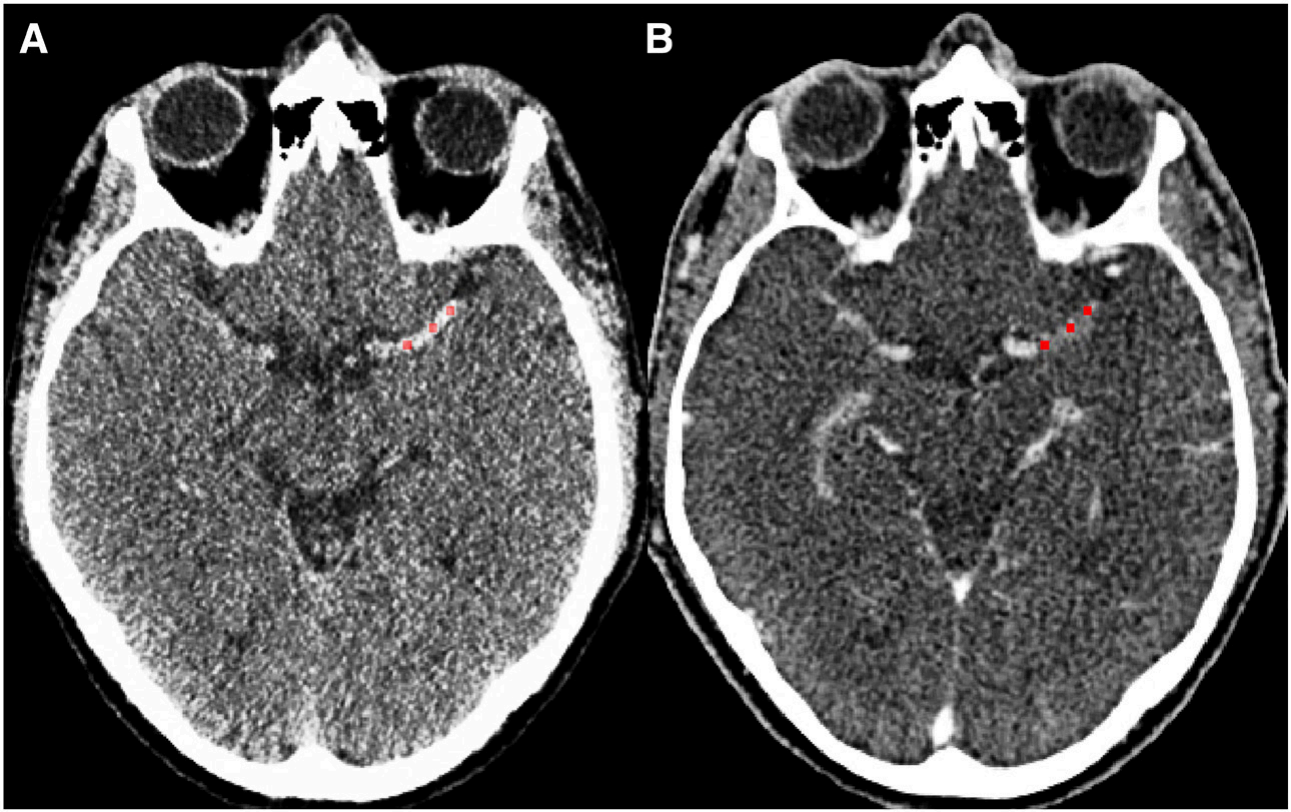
Thrombus attenuation increase measurement. Three regions of interest (red) are placed in a left middle cerebral artery thrombus on noncontrast computed tomography (CT; A) and CT angiography (B). To calculate thrombus attenuation increase as a measure for perviousness, the density (in Hounsfield Units) in each region of interest is measured. Subsequently, the average noncontrast CT attenuation value is deducted from the average CT angiography value.
